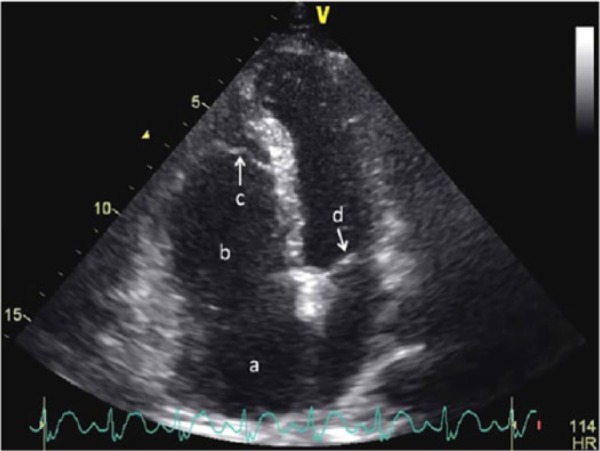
Echocardiography (apical 4-chamber view) showing features of Ebstein anomaly (a, right atrium; b, dilated atrialized portion of right ventricle; c, downward displacement of tricuspid valve; d, mitral valve).
